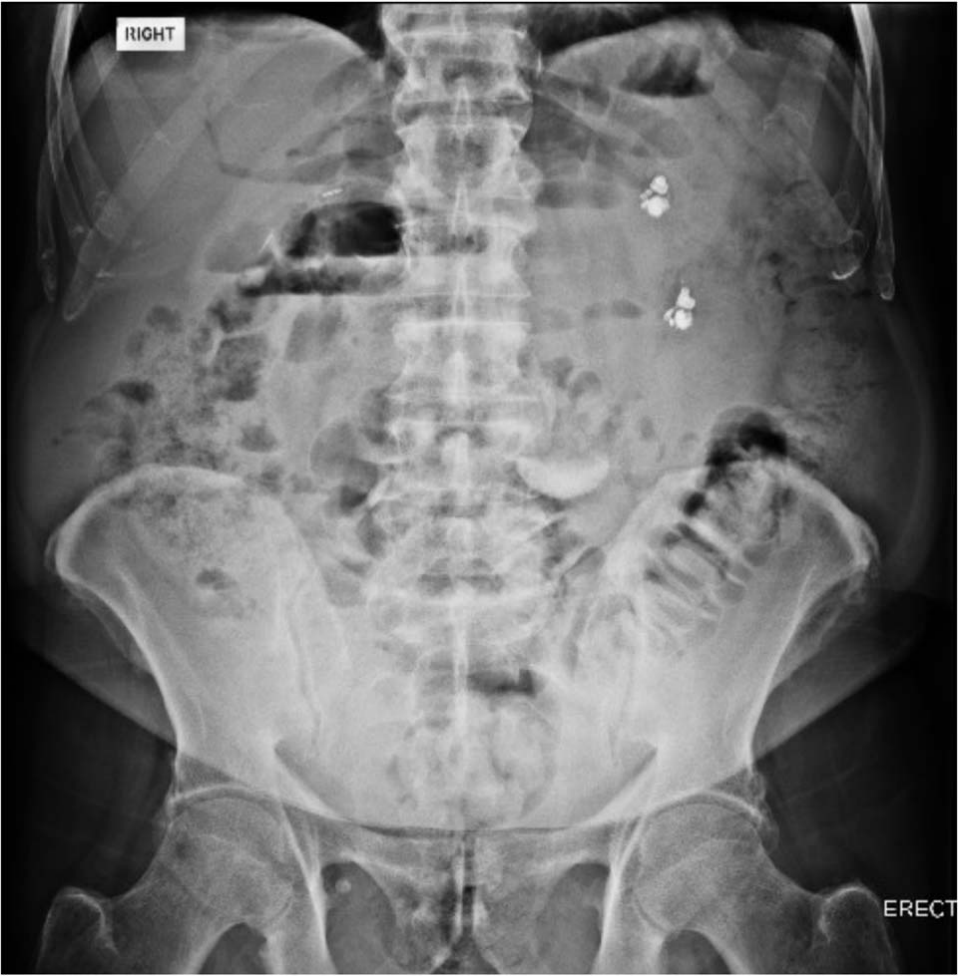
Abdominal X-ray identifying two hearing aids on day of initial presentation.
